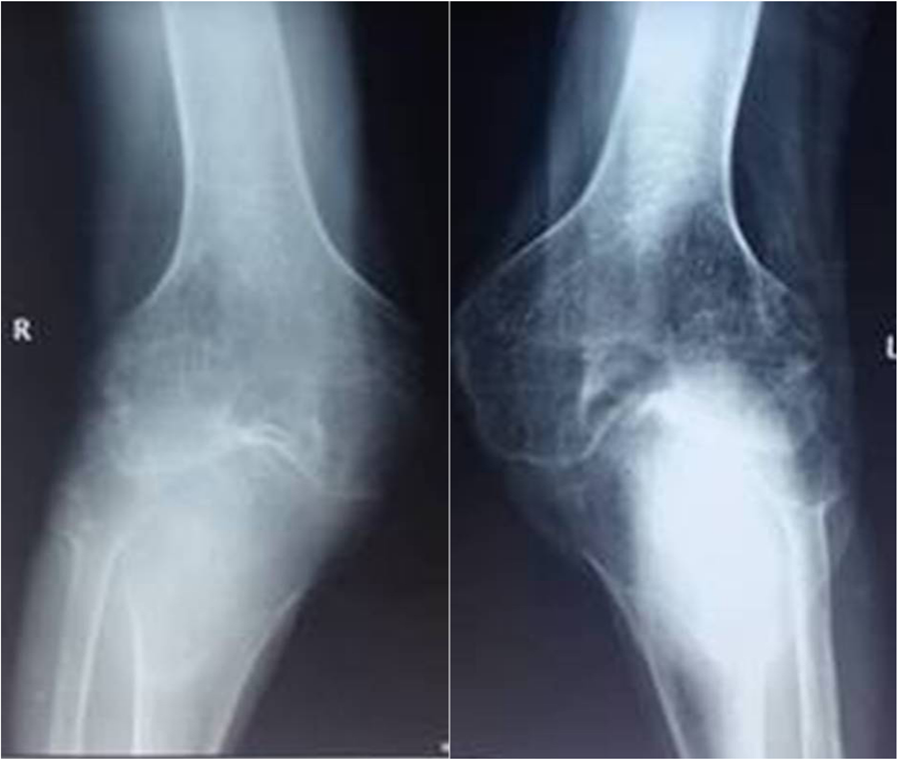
Preoperative anteroposterior radiographs of both knees showing complete complete disappearance of the joint spaces
